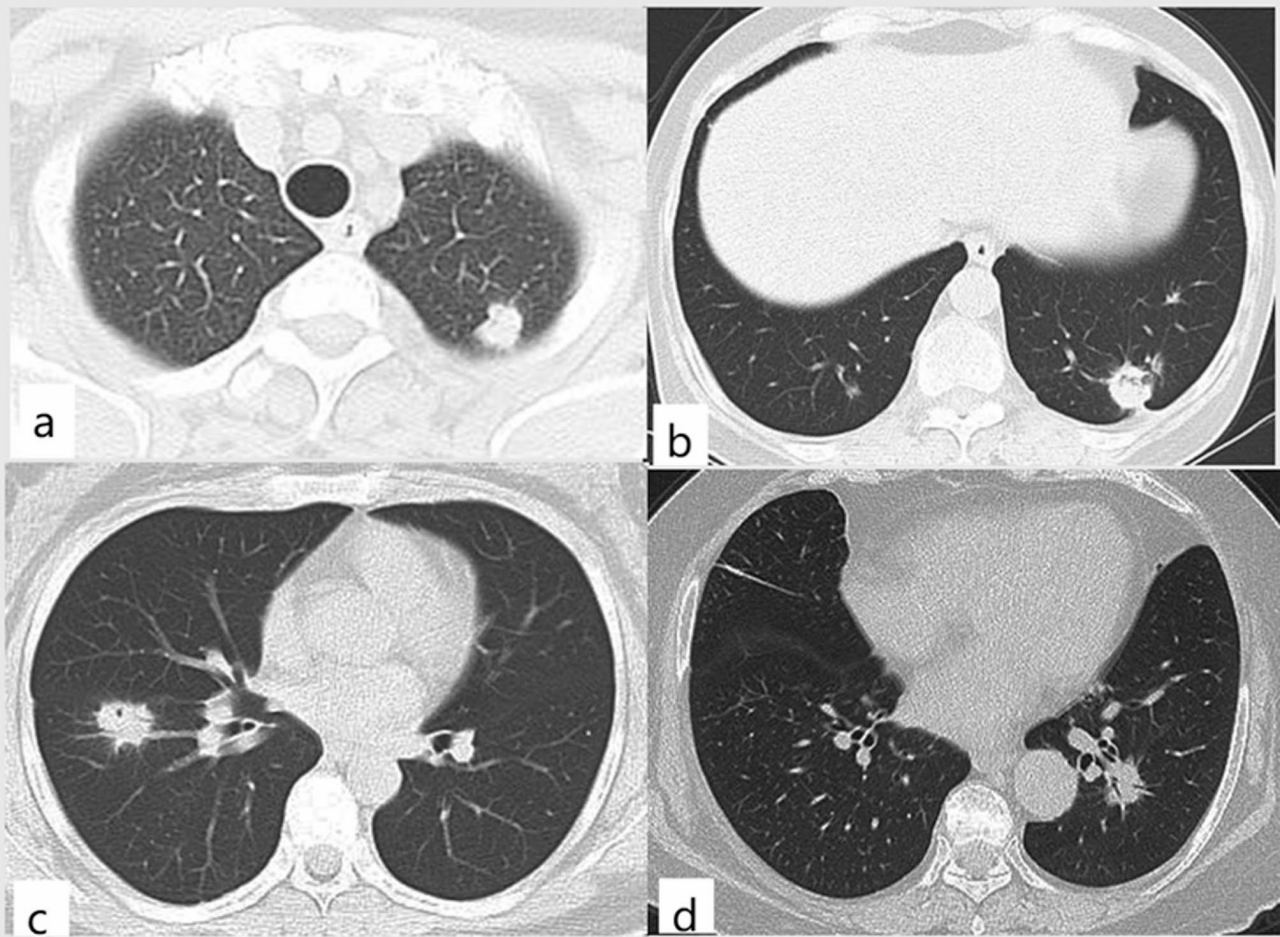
CT findings of nodule-mass PC. Axial CT image showed a lobulated nodule in the subpleural region of the left upper lobe (a). Axial CT image showed a nodule with vacuole, spiculation, and pleural indentation in the left lower lobe (b). Axial CT image showed a nodule with vacuole and spiculation in the right lower lobe (c). A nodule with spiculation and air-bronchogram could be seen in the left lower lobe on axial CT (d)
